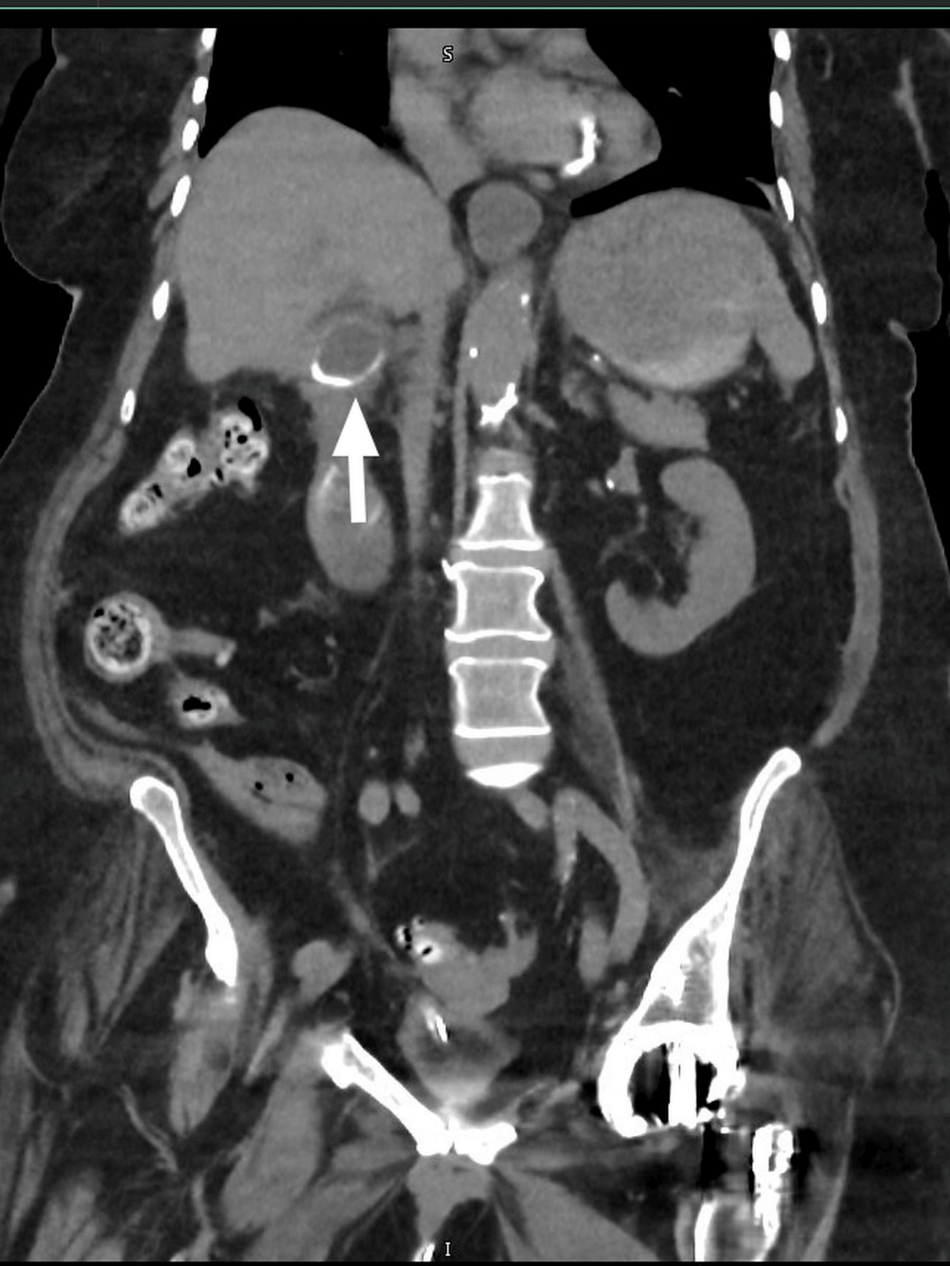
Coronal image Coronal CT image of the abdomen showing a large 3.3 cm calcified gallstone (white arrow) impacted at the gallbladder neck. The gallbladder appears collapsed, and there is associated pneumobilia, supporting the presence of a cholecystoenteric fistula.
